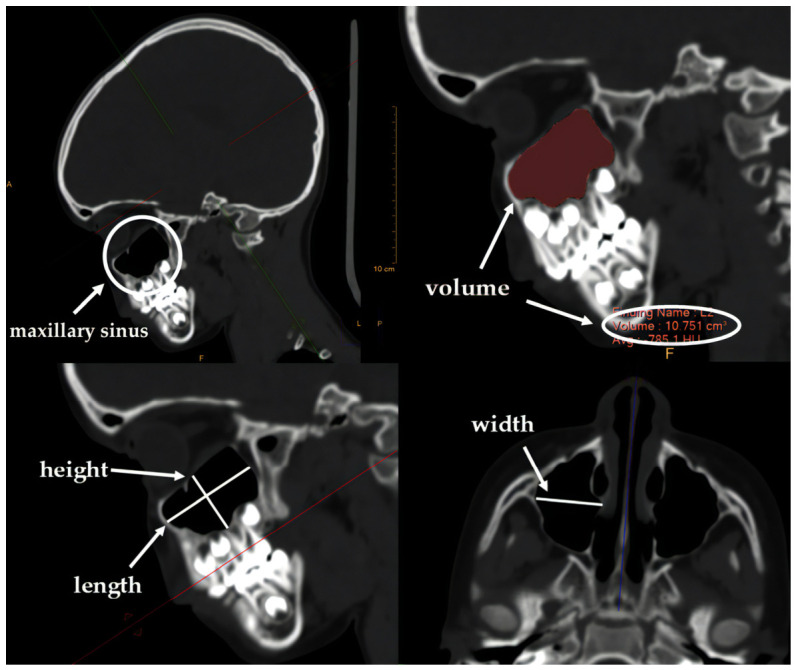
Head computed tomography in children showing the maxillary sinus measurements used in the study: volume, height, length, and width.
